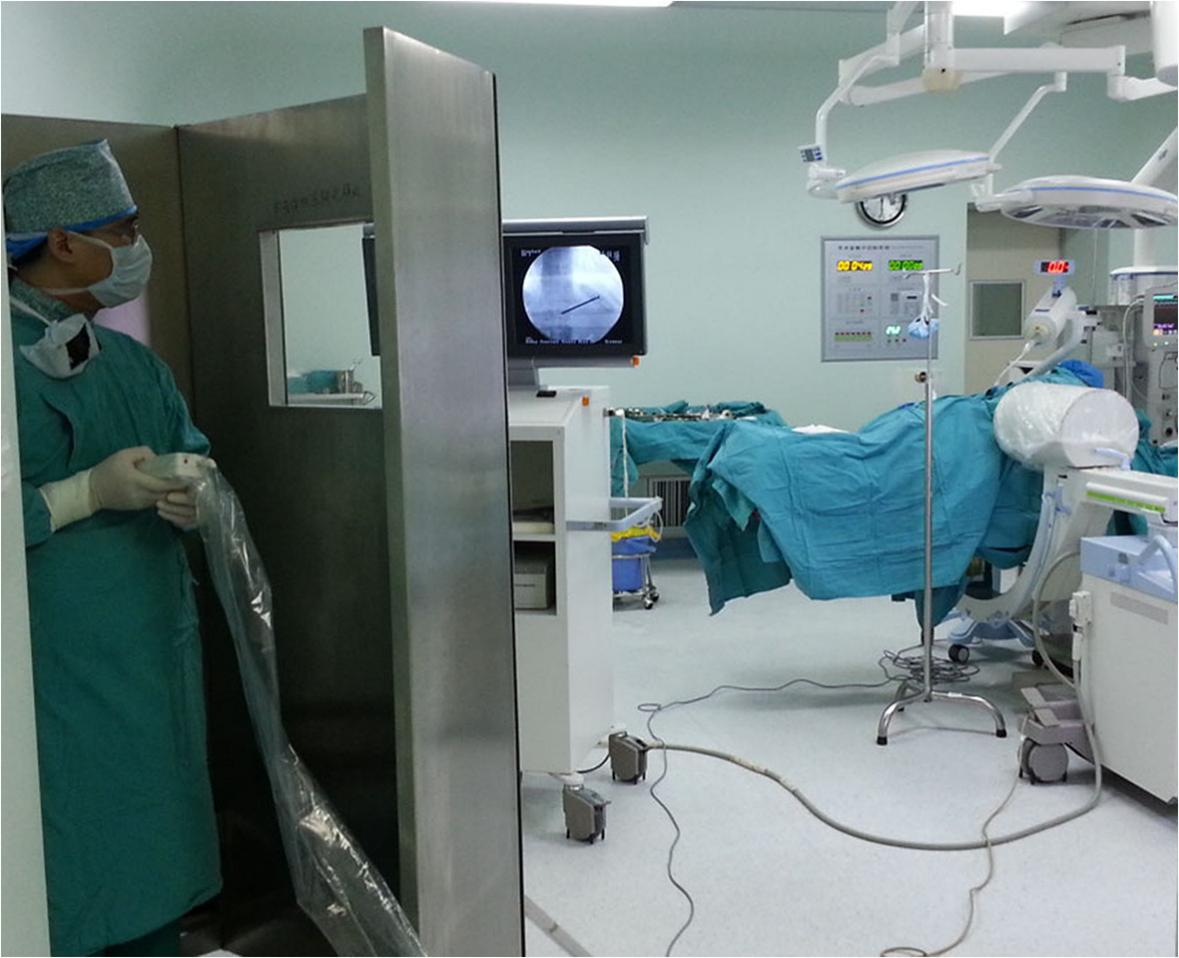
The operator stood behind the lead sheet about 4 meters away from X-ray tube and injected cement through the forward key on the handheld controller (it can be wraped by steriled sheath).
